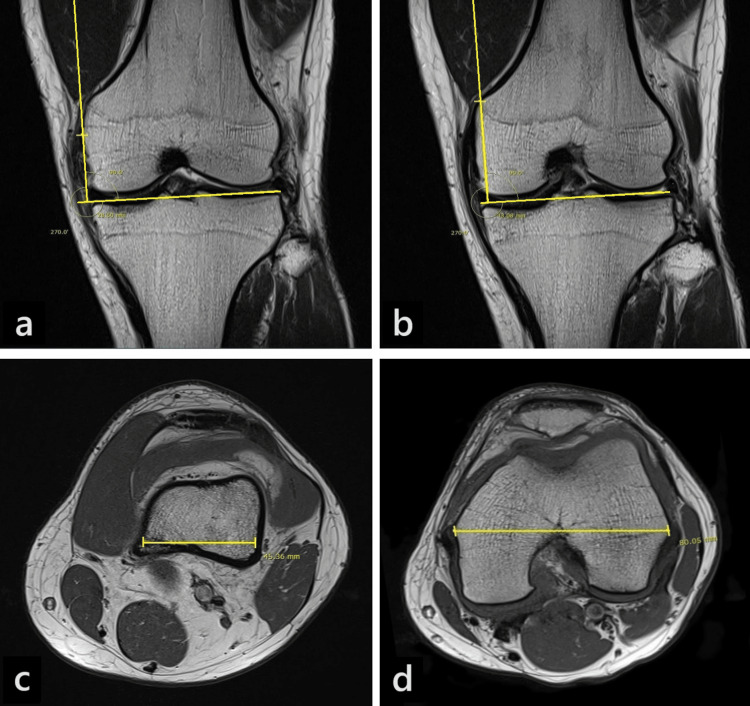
Measurements on the MRI scans a) Measurement of MEJL on coronal slice 1; b) Measurement of ATJL on coronal slice 2; c) Measurement of ATW on axial slice 1; d) Measurement of TEW on axial slice 2. MEJL: medial epicondyle to joint line distance, ATJL: adductor tubercle to joint line distance, ATW: width of the femur at the level of the adductor tubercle, TEW: transepicondylar width
